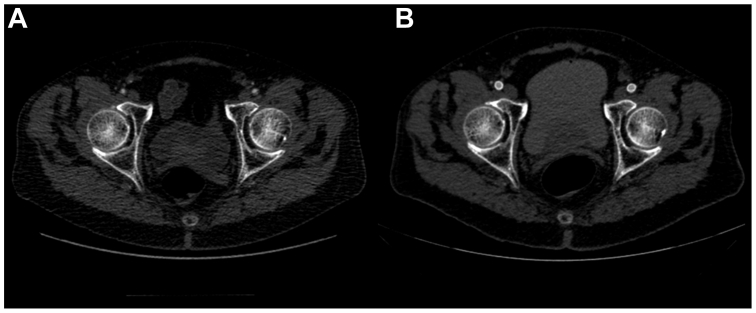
A, Computed tomography angiography (CTA) reveals a hostile anatomy for complex endovascular aortic aneurysm repair (EVAR) or thoracic endovascular aortic aneurysm repair (TEVAR. B, CTA reveals endoconduit (EC) placement to improve the future access site during complex EVAR or TEVAR.
